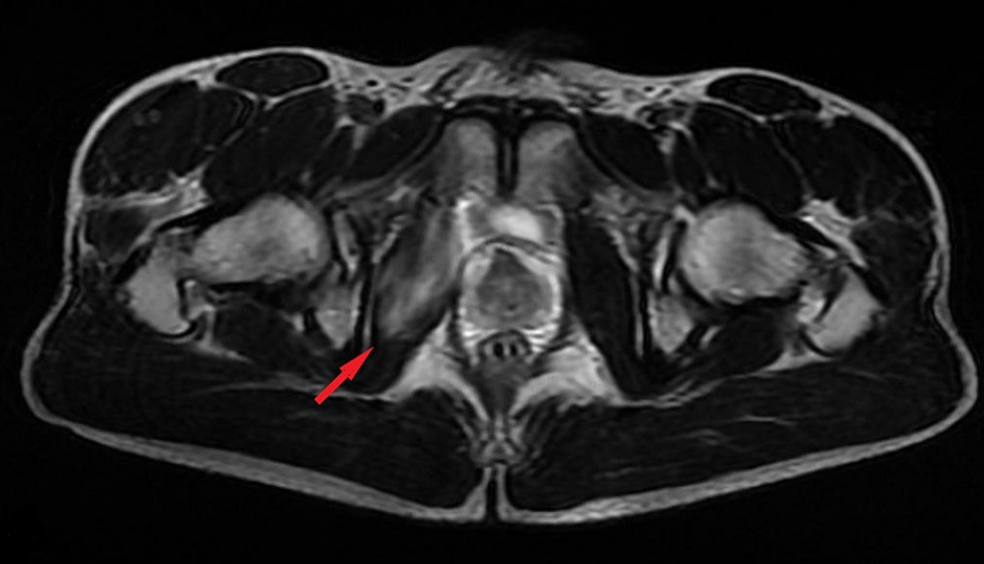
MRI T2 Sequence Axial View FSE Axial view of the right hip at the level of greater trochanter. Hypersignal (Grade II muscle strain) in the obturator internus at the intrapelvic route, with the development of edema and fluid collections around and between its fibers. No other pathology was revealed. The red arrow shows the obturator internus. FSE: Fast Spin Echo
